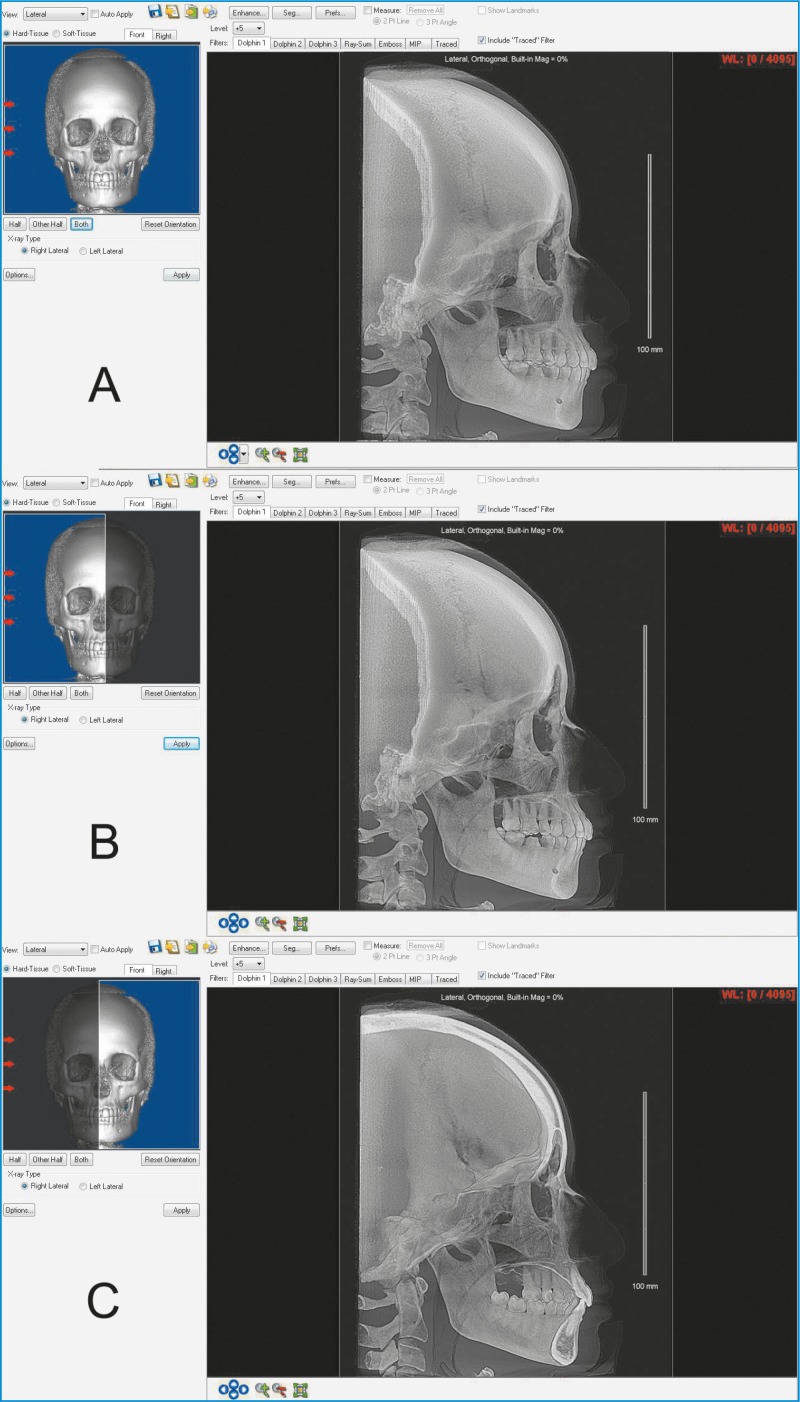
Different modalities of CBCT-derived cephalograms from the same subject A)
